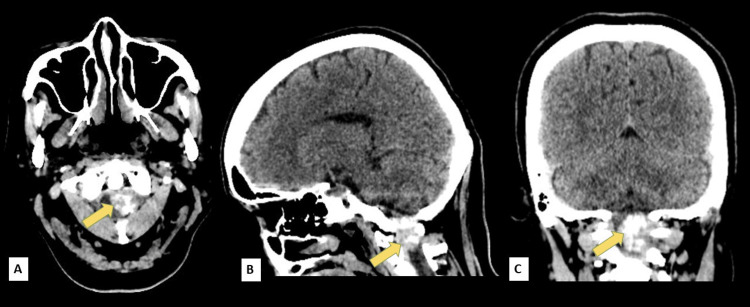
Plain cranial computed tomography (CT) scan in (A) axial, (B) sagittal, and (B) coronal views showing a well-defined extra-axial hyperdense mass with intralesional calcifications measuring 3.2x2.0x1.9 cm (CCxWxAP) within the spinal canal at the level of C1-C2 (arrow) CC: Craniocaudal; W: Width; AP: Anteroposterior
